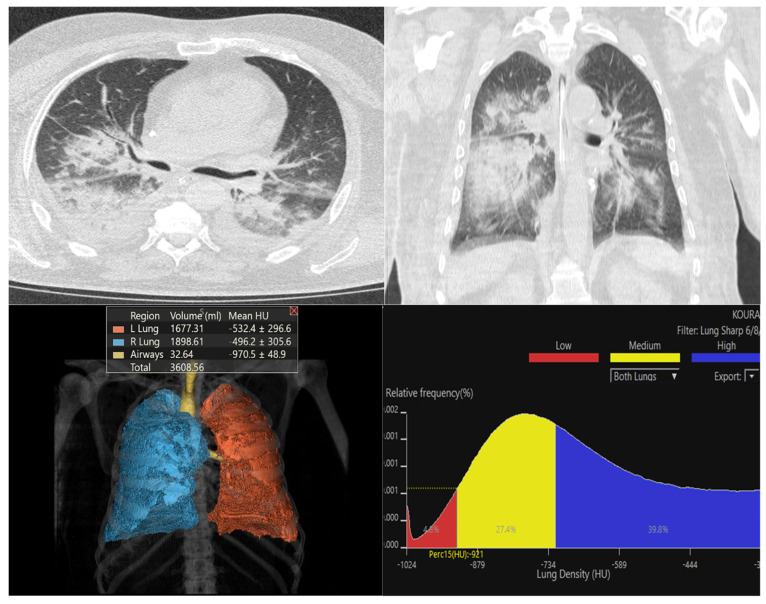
Quantitative analysis of a case presenting with bilateral but right-dominant asymmetric parenchymal involvement. The CT sections in the upper panel reveal diffuse ground-glass opacities and patchy consolidation in both lungs, with the pathology being visibly denser in the right hemithorax. Quantitative data in the lower left quadrant corroborate this visual asymmetry; the mean density of the right lung (mean HU: −496.2 HU) is markedly higher than that of the left lung (mean HU: −532.4 HU). The histogram analysis in the lower right shows a heterogeneous density distribution, where high-density areas (blue, 39.8%) and medium-density transition zones (yellow, 27.4%) predominate over normal parenchyma.
